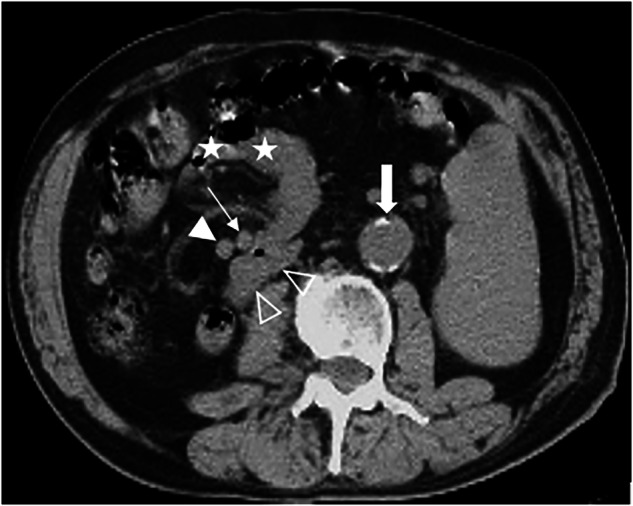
Eighty-one-year-old man with jejunal transposition. Axial CT scan showed the obvious tortuosity and distance between the SMA (thin arrow) and aorta (thick arrow), with the horizontal segment of the duodenum (hollow triangle) traversing normally behind the SMA, the normal relationship of SMA/SMV (solid triangle), the DJJ and jejunum (star) positioned in the right abdomen, forming a reverse “C” shape
